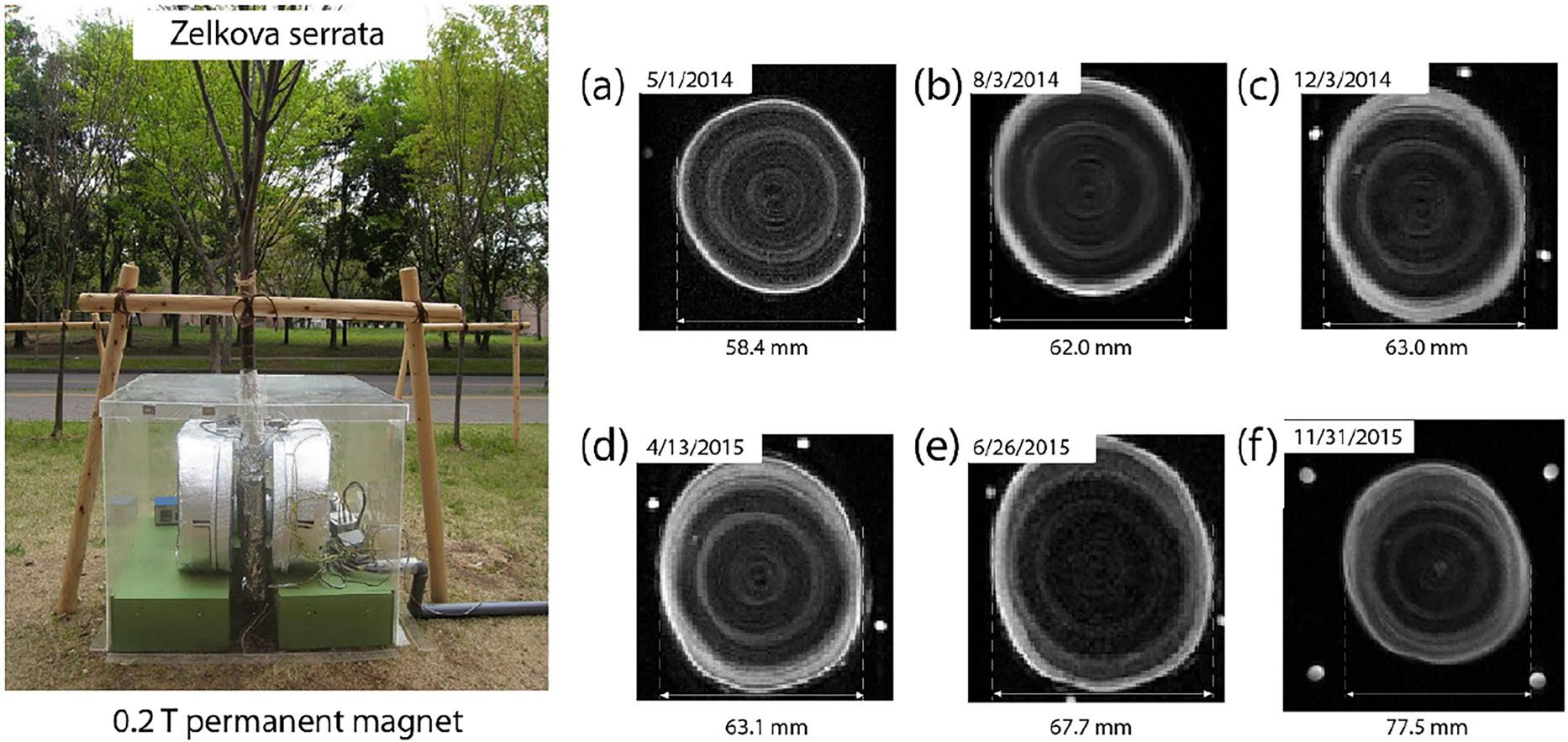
0.2 T-MRI positioned outside the laboratory on the stem of *Zelkova serrata*. Images (a–f) shows the growth of the stem, an increase of the stem diameter being observed (Adapted from [33] with permission from Elsevier)
